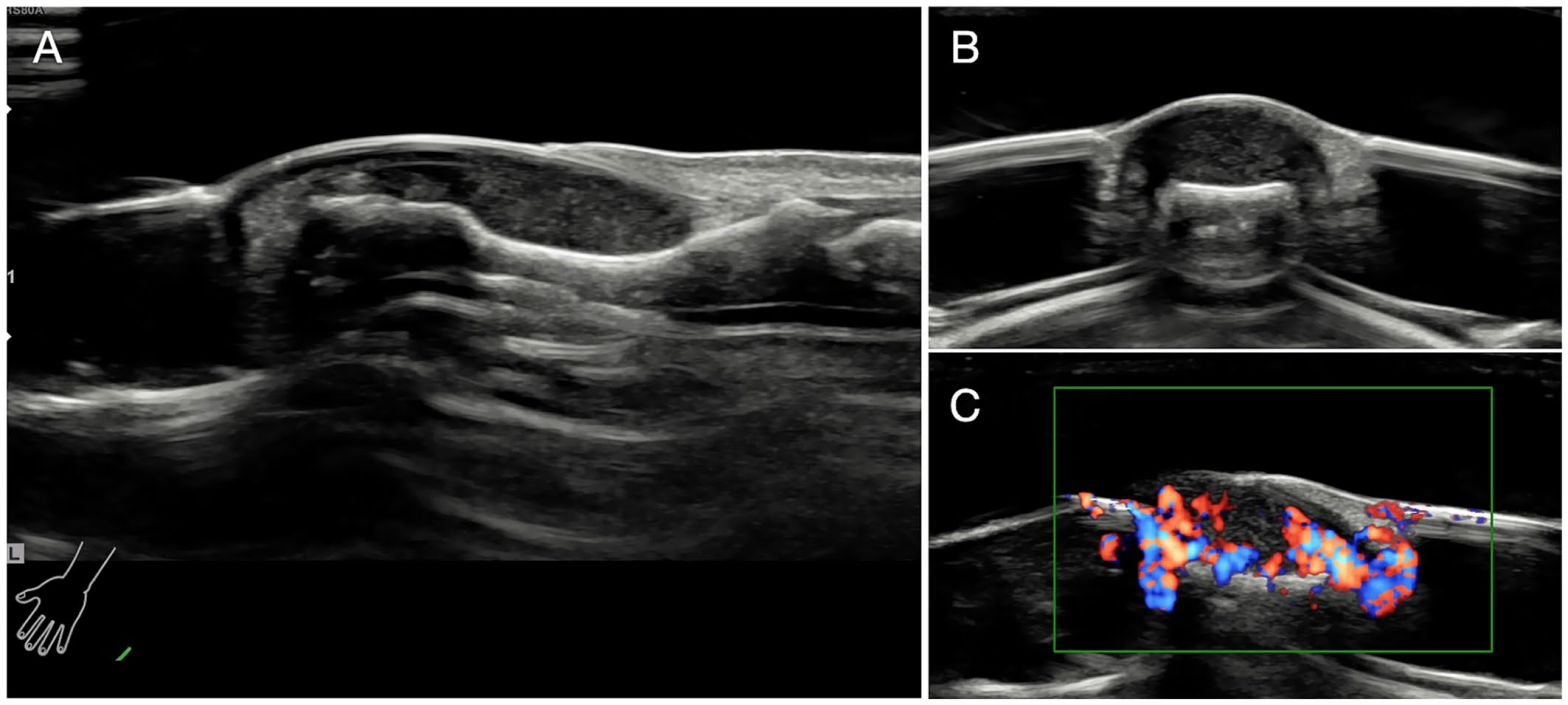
Ultrasonic investigation. (A) Glomus tumor exhibited regular shape and clear margin under ultrasonic examination. Tumors were low-echo without lateral shadows locating in the subungual area. (B) The ultrasonographic view of coronal section of the affected finger. (C) Abundant blood flow and vessels in and out of the tumors were observed.
